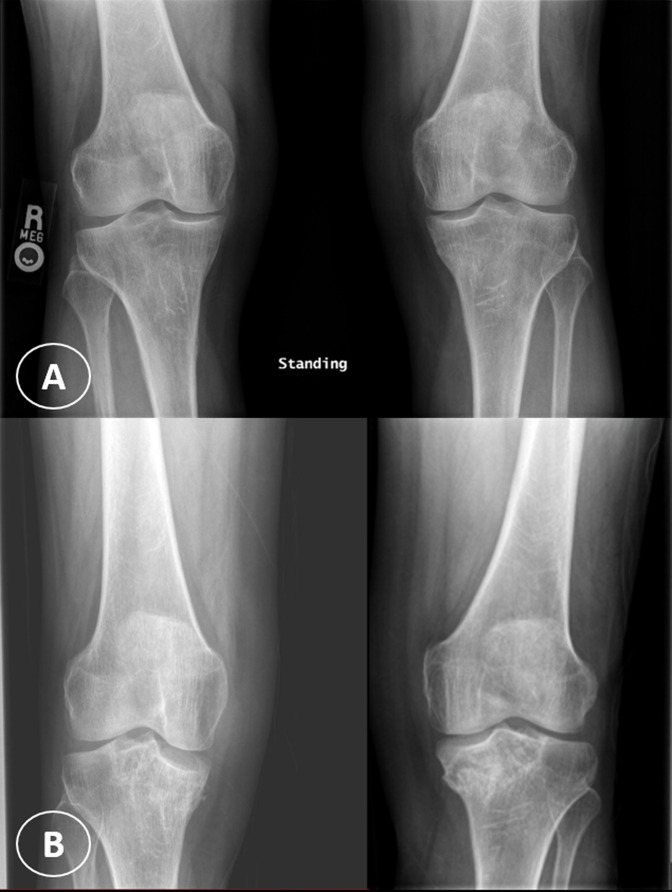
Anteroposterior plain radiograph of both knees done at the initial presentation with normal appearance (A) and at 3 months afterward (B) demonstrating bilateral subchondral sclerotic lesions and collapse of the medial tibial plateau more severe in the right knee.
